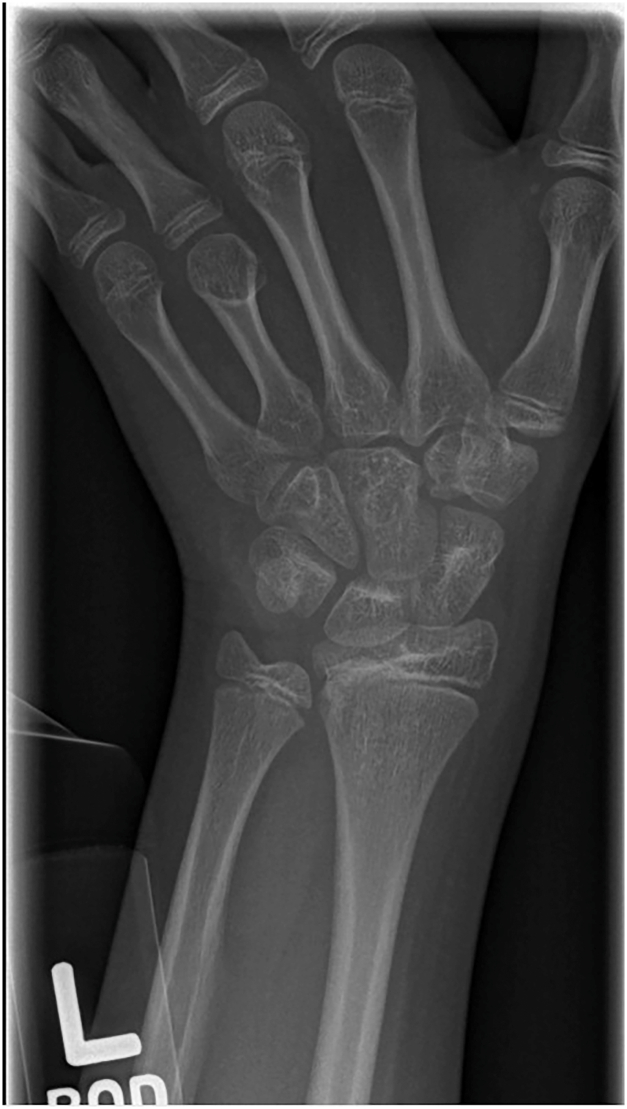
Radiograph of the left hand, demonstrating a short fourth metacarpal.
